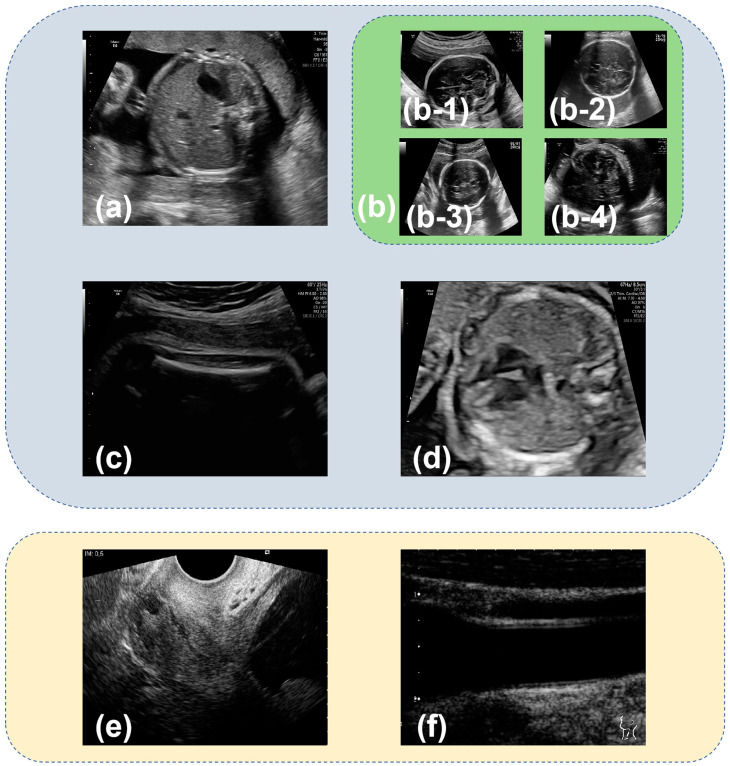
Representative images from Burgos-Artizzu’s dataset [22] depicting various fetal ultrasound standard planes: (a) fetal abdominal standard plane; (b) fetal brain standard plane; (b-1) fetal trans-ventricular standard plane; (b-2) fetal trans-thalamic standard plane; (b-3) fetal trans-cerebellum standard plane; (b-4) other brain standard plane; (c) fetal femur standard plane; (d) fetal thorax standard plane; (e) maternal cervix; and (f) other. (a–f) are samples for six categories classification. (a–f) are samples for nine categories classification.
